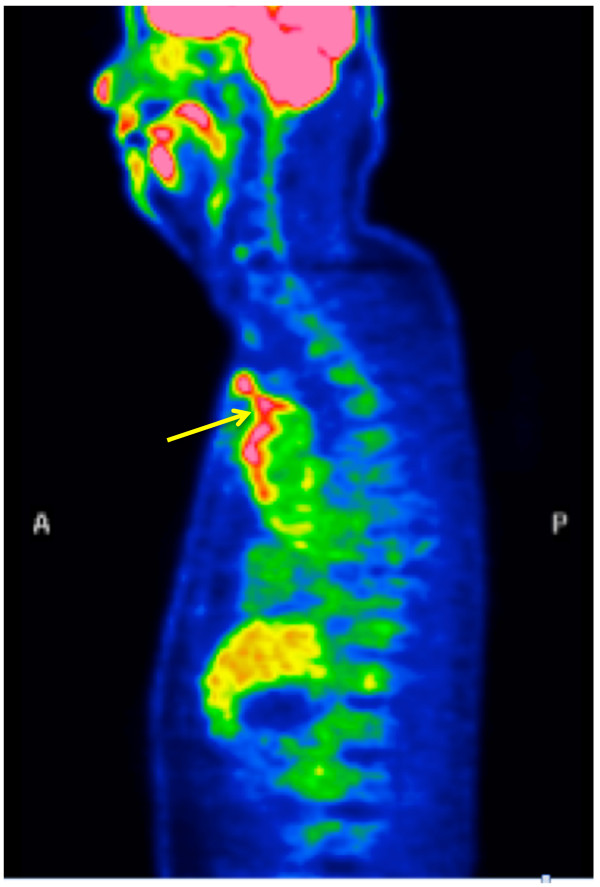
^18^ F-FDG-PET/CT in Case 2.*Arrow*; Abnormal ^18^ F-FDG accumulation is extensive around the ascending aortic graft (SUVmax 6.22), continuous from the upper edge of the graft to the fistula.
